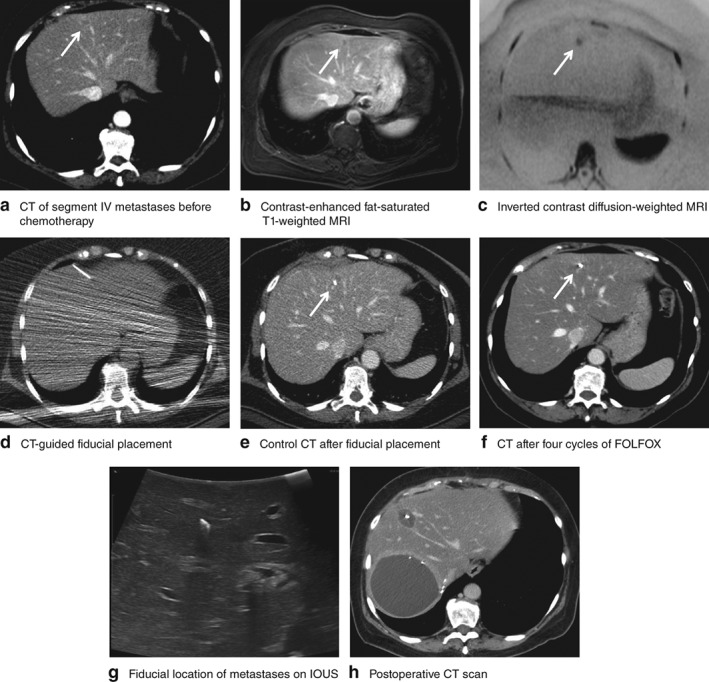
Left colonic adenocarcinoma with four liver metastases in segments IV, VI, VII and VIII. Before chemotherapy, cross‐sectional images from a CT, b MRI contrast‐enhanced fat‐saturated T1‐weighted image and c MRI inverted contrast diffusion‐weighted image showed liver metastasis at risk of being missed (white arrow) in segment IV. After CT‐guided marking (d) a control CT scan confirmed good fiducial marker placement (e). f After four cycles of FOLFOX–bevacizumab, the marked lesion disappeared from the CT scan. g The fiducial marker allowed the location of the missing metastasis to be identified easily by intraoperative ultrasonography (IOUS), allowing radiofrequency ablation in addition to a right hepatectomy. h A postoperative control CT scan confirmed the good targeting of the ablation
